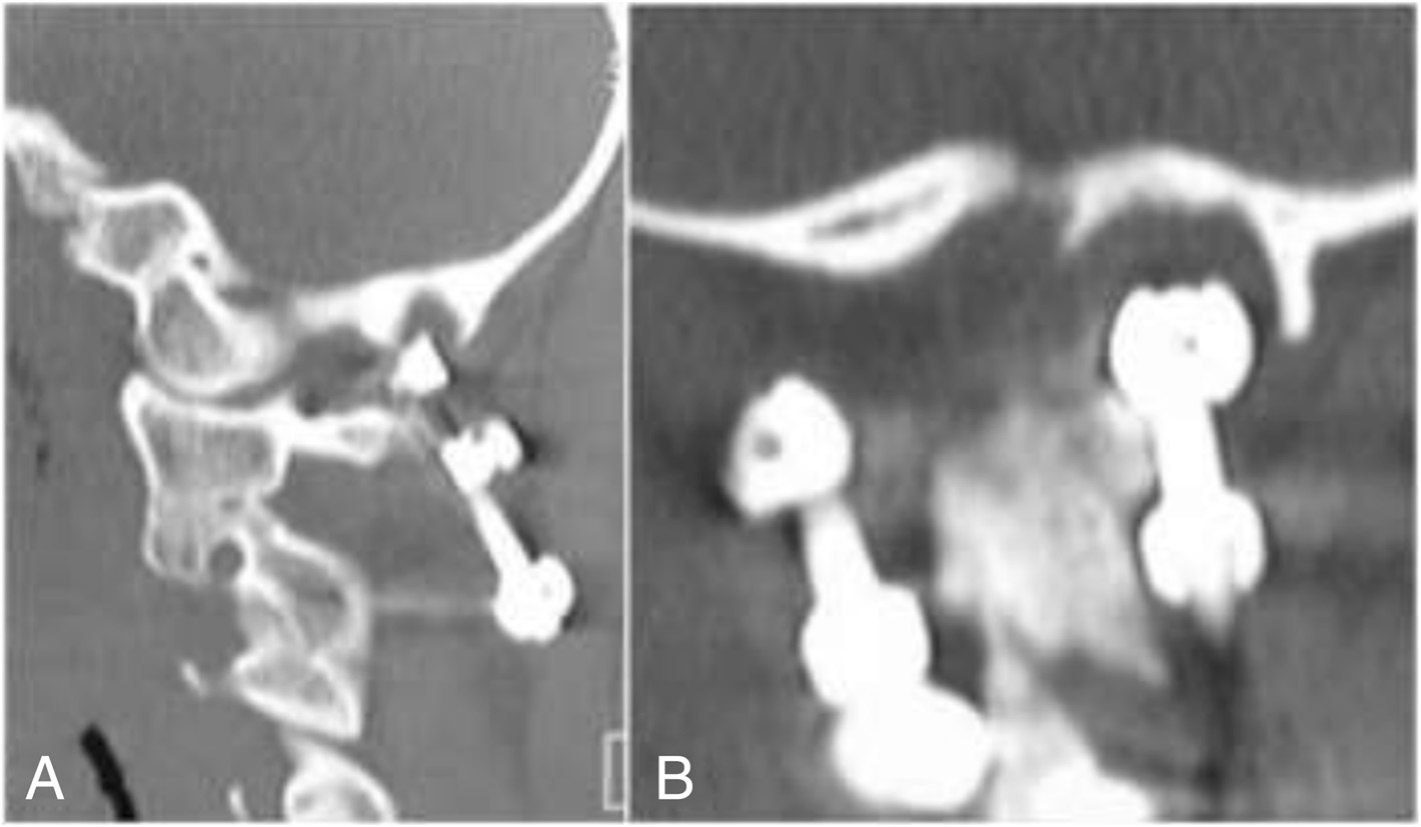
MPR-CT images of a 14-year-old boy obtained 8 months following C1-C2 fusion using a C1 hook and C2 laminar screw. A. The sagittal image shows that the supralaminar hook on the left protrudes into the occipital bone. B. The coronal image shows that the occipital bone is eroded by the supralaminar hook on the left.
